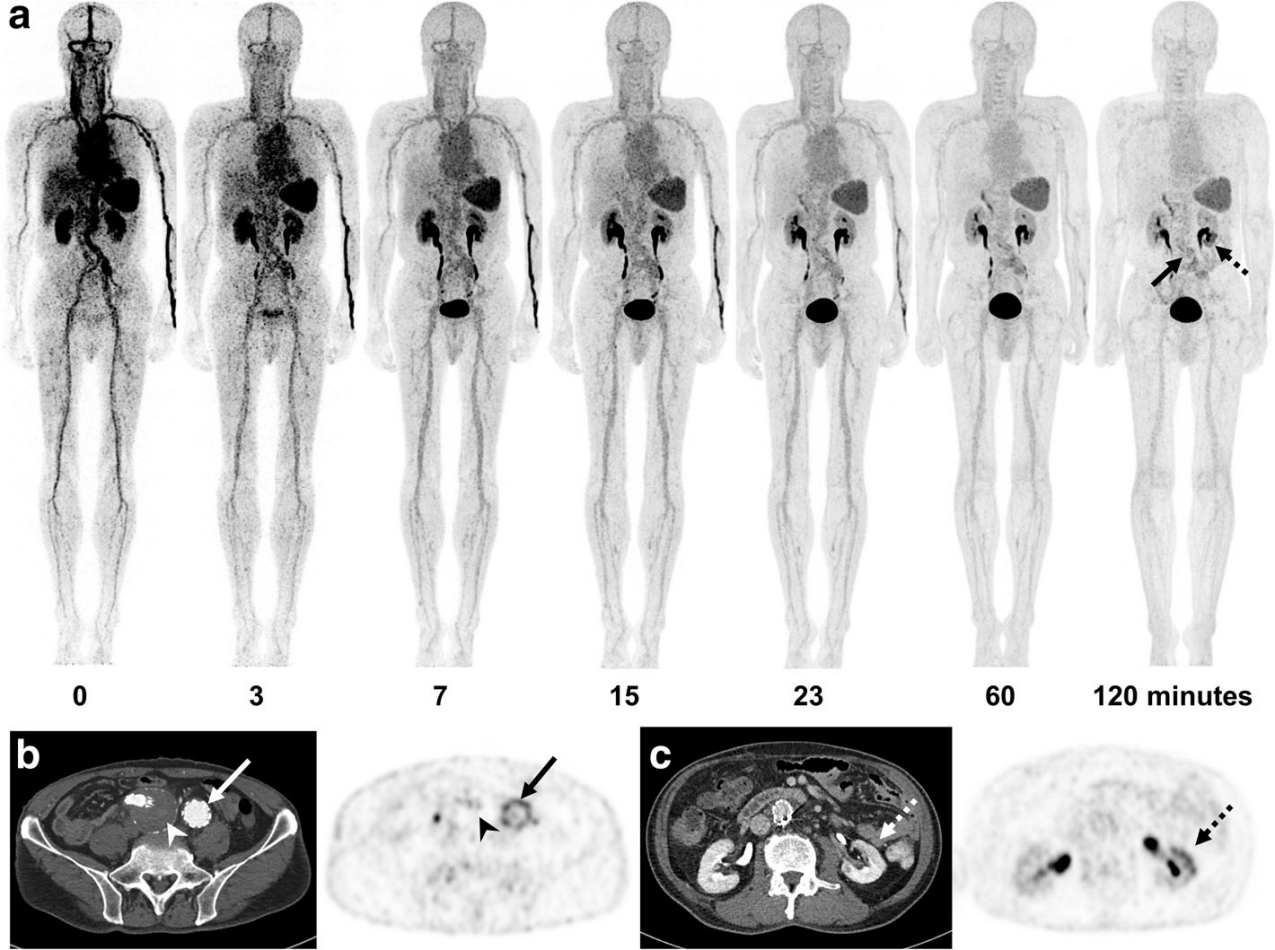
^18^F-GP1 PET/CT and CT images of a 73-year-old man who had undergone endovascular abdominal aortic aneurysm repair surgery. Anterior maximum intensity projections of ^18^F-GP1 PET/CT show positive ^18^F-GP1 accumulation in the inner surface of abdominal aortic graft (arrow) and the lower portion of the left kidney (dotted arrow), which are increasingly distinct in later images as background ^18^F-GP1 activity clears over time via urinary and hepatobiliary excretion (a). Transaxial CT and PET images show increased ^18^F-GP1 uptake in the inner surface of the left iliac artery graft (b, arrows), but no ^18^F-GP1 uptake in the chronic intraluminal thrombus in the right iliac aneurysmal sac (b, arrow heads). Additional positive ^18^F-GP1 uptake is observed in the non-enhancing renal ischaemic area involving the lower portion of the left kidney (c, dotted arrows)
